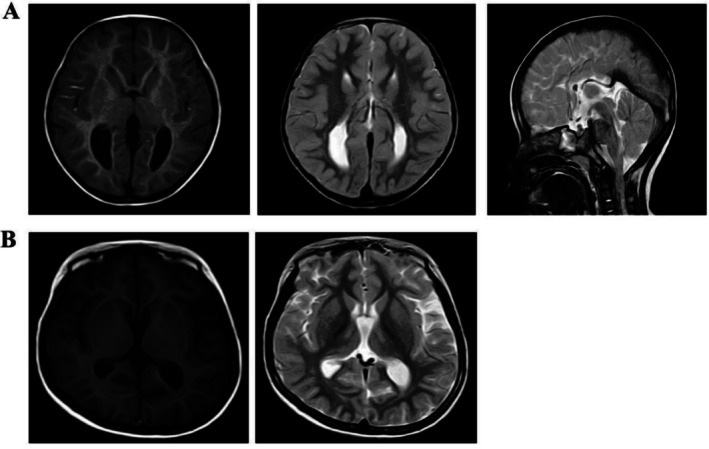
(A) An MRI of the brain of Case 1 showing congenital absence of a corpus callosum. (B) A brain MRI of Case 2 suggests cerebral atrophy and dilated supratentorial ventricles.
